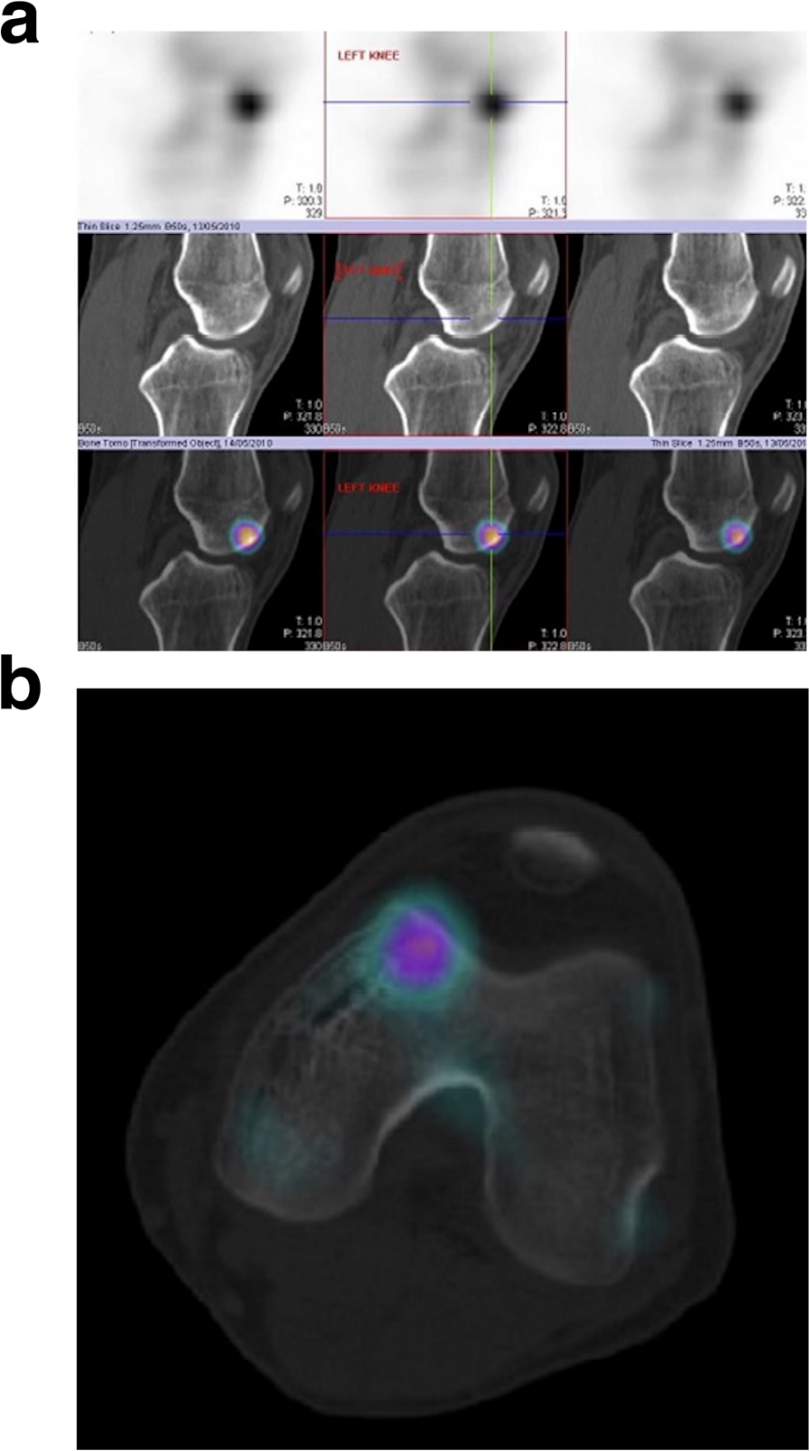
a Sagittal SPECT-CT scan slices of the same patient demonstrating obvious abnormality with focally increased tracer activity pertaining to the sub-chondral aspect of the medial femoral condyle. b Tranaxial SPECT-CT slice of the left knee highlighting the lesion on the medial femoral condyle
